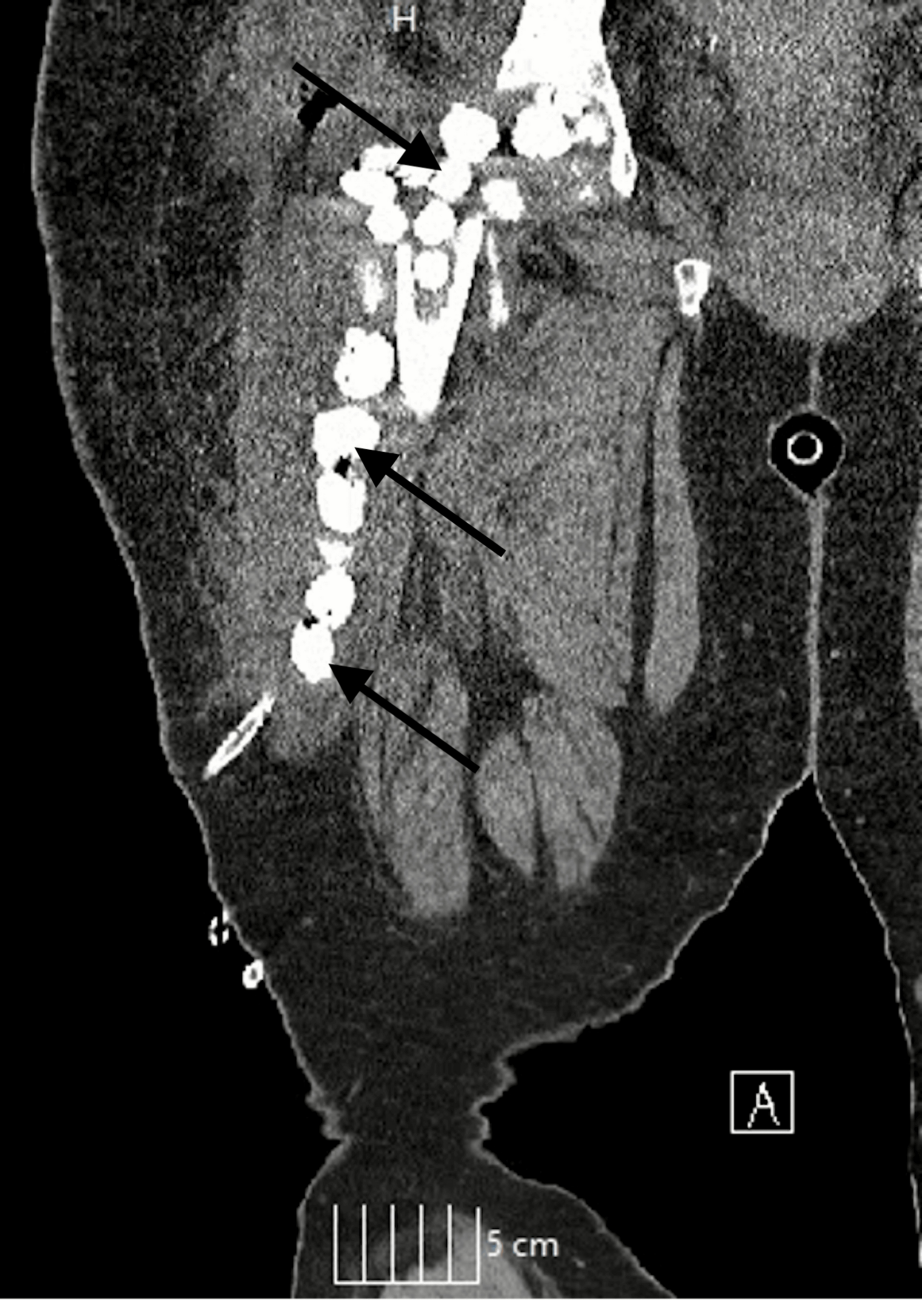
Postoperative CT scan - removal of hardware and osteotomy of the proximal femoral head and neck, displaying multiple intramuscular echinococcal cysts versus multiple abscesses
